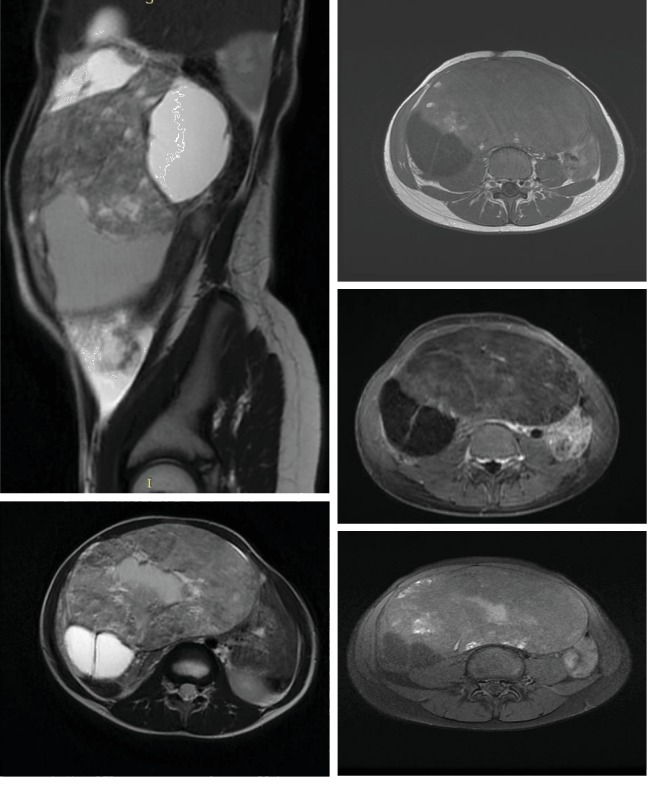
Axial T1-weighted, axial contrast-enhanced fat-suppressed T1-weighted, axial T2-weighted, sagittal T2-weighted, and contrast-enhanced T1-weighted magnetic resonance (MR) images demonstrate a solid cystic mass of approximately 126 mm in diameter in the abdominopelvic cavity. The cystic structures are of different signal, and the solid mass is raised discretely after injection of contrast medium, probably originating from the left ovary.
